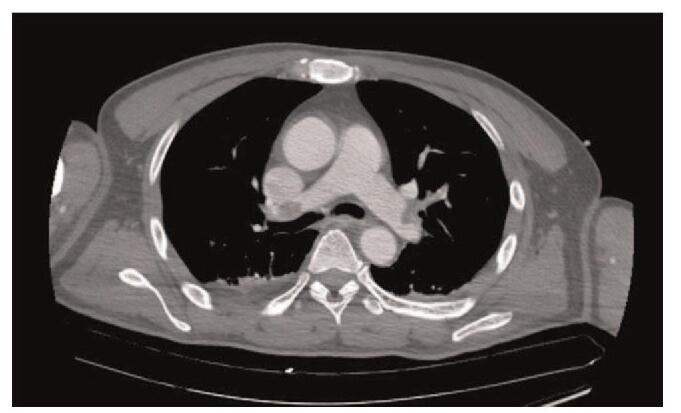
Chest computed tomography showing the emboli in both pulmonary arteries.
